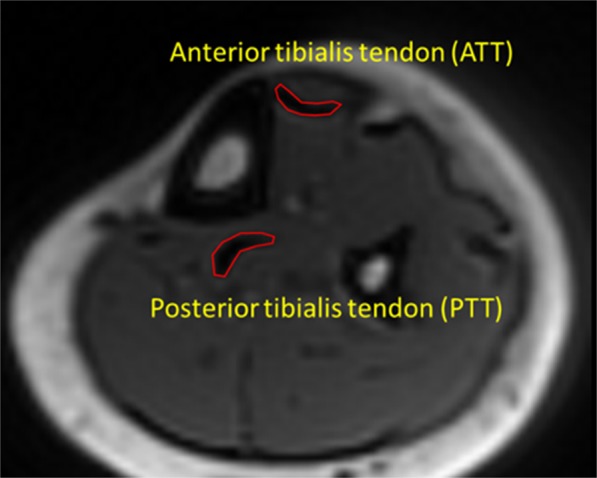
A representative Cones UTE-MRI image from a 50-year-old female subject (TR = 20 ms and TE = 2 ms). Tibialis anterior and posterior tendons were obvious in the MRI images, and are indicated in red contours.
